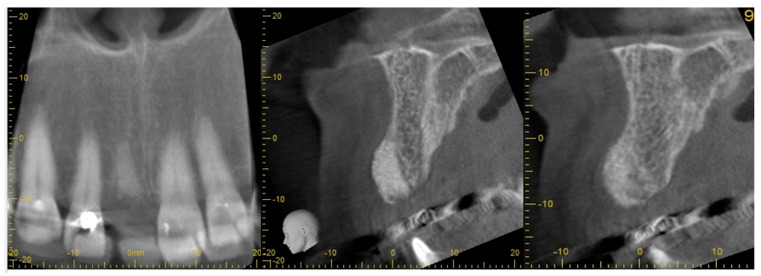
Extraction of UR1 and ARP using BioOss^®^ and A-PRF. A CBCT was taken 8 weeks after ARP showing excellent maintenance of alveolar bone contour with radiologically dense bone.
